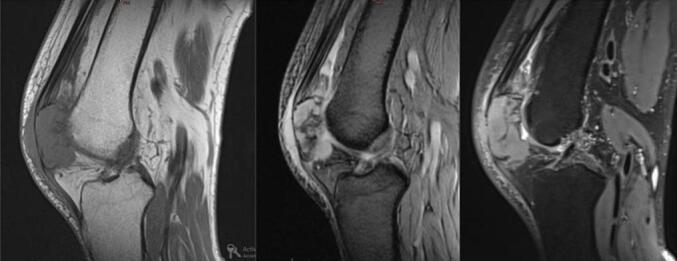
MRI findings:* Marrow replacement with low T1 signal intensity, high STIR signal, marked contrast enhancement, cortical disruption, and adjacent soft tissue oedema.
